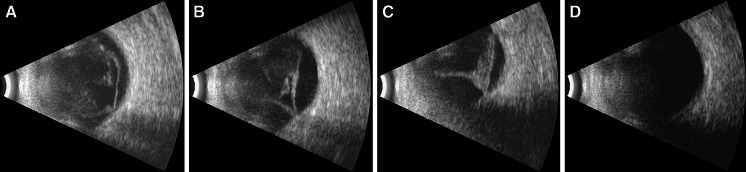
Consecutive ultrasound scans, showing gradual thickening of vitreous exudate and posterior vitreous detachment (a–c) and clear vitreous chamber after pars plana vitrectomy (d)
